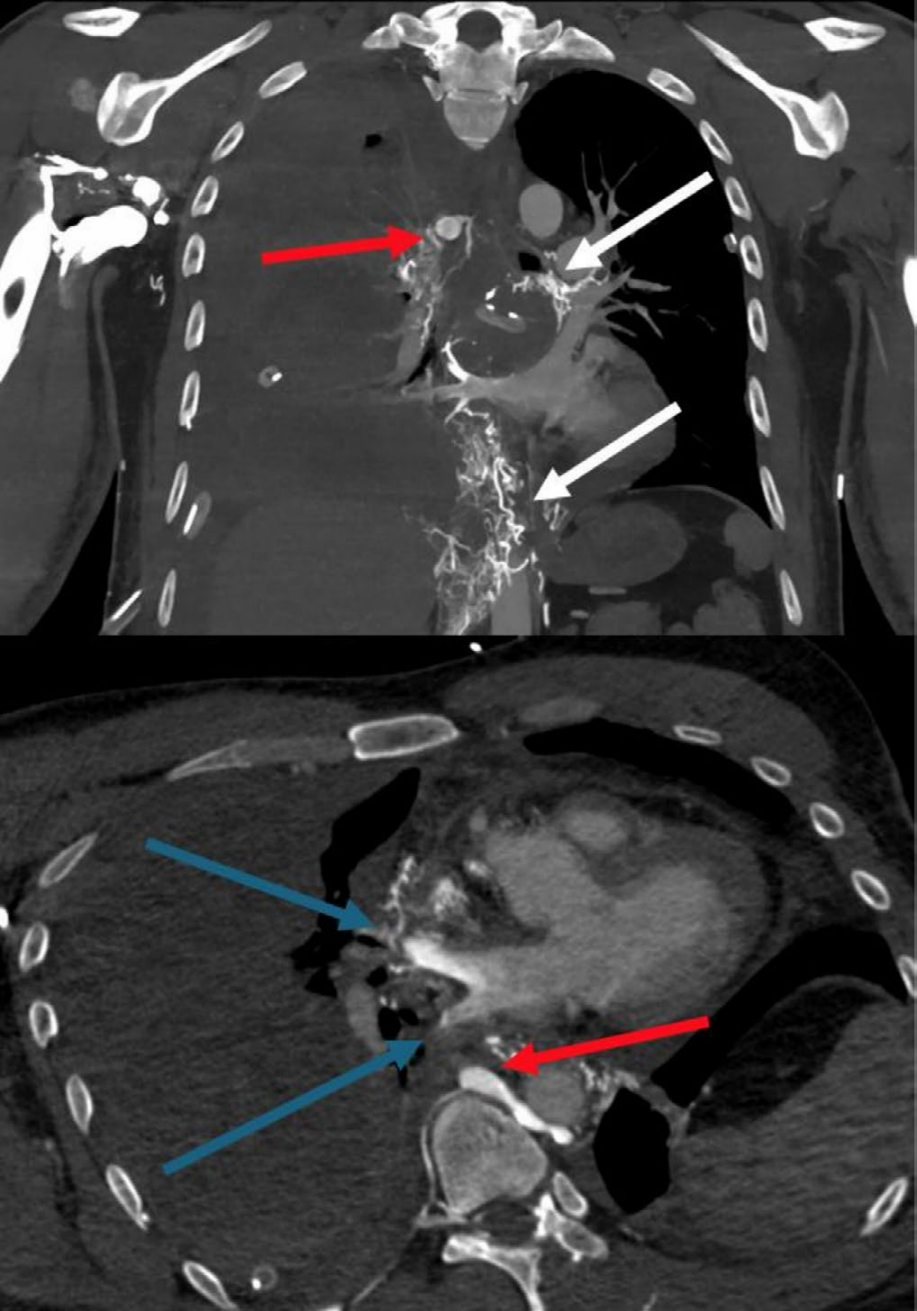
Contrast‐enhanced CT showing (red arrows) recruitment of the azygos system and (white arrows) a pericaval cavernous transformation connecting the upper limb venous system and the pulmonary veins, with (blue arrows) early venous opacification of the pulmonary vein through mediastinal anastomosis. Complete right lung atelectasis is secondary to hemothorax induced during the initial management strategy with therapeutic anticoagulation.
